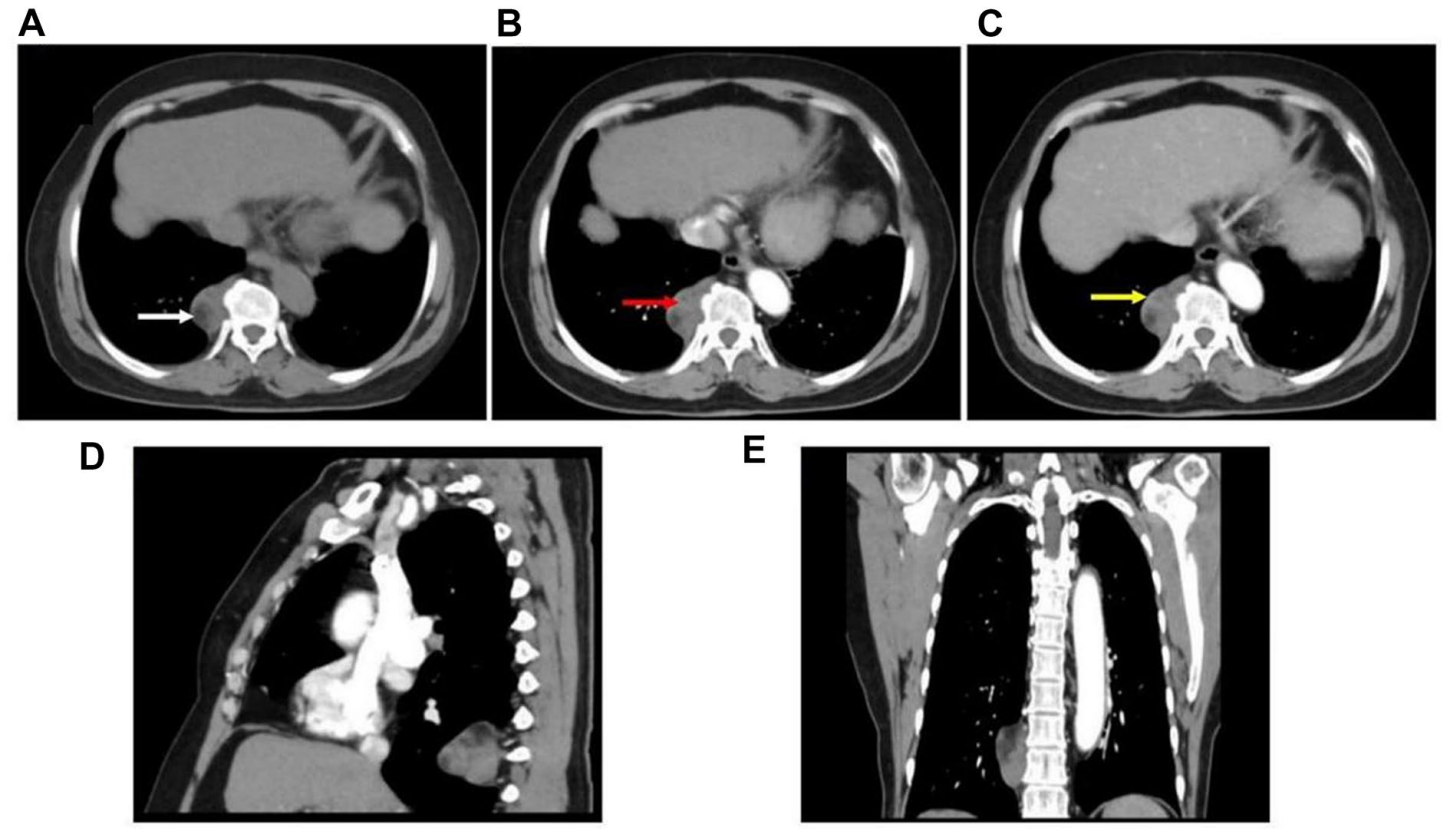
CT image of a right posterior mediastinal myelolipoma: The white arrow in (A) shows the fat composition; the red arrow in (B) shows mild enhancement (the common CT value in this area is 45Hu, 58Hu in arterial phase, and 67Hu in venous phase). The yellow arrow in (C) shows the capsule
